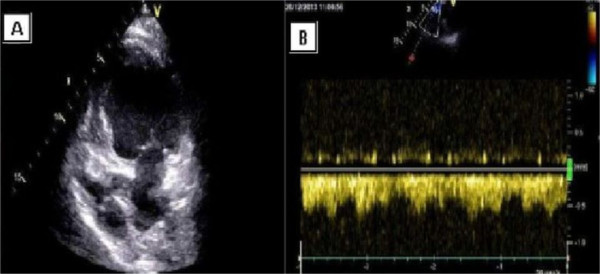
Transthoracic echocardiography. Panel A: Apical four chamber view showing tricuspid atresia. Panel B: Doppler interrogation of the anastomosis (superior vena cava to right pulmonary artery) revealed a laminar pattern.
